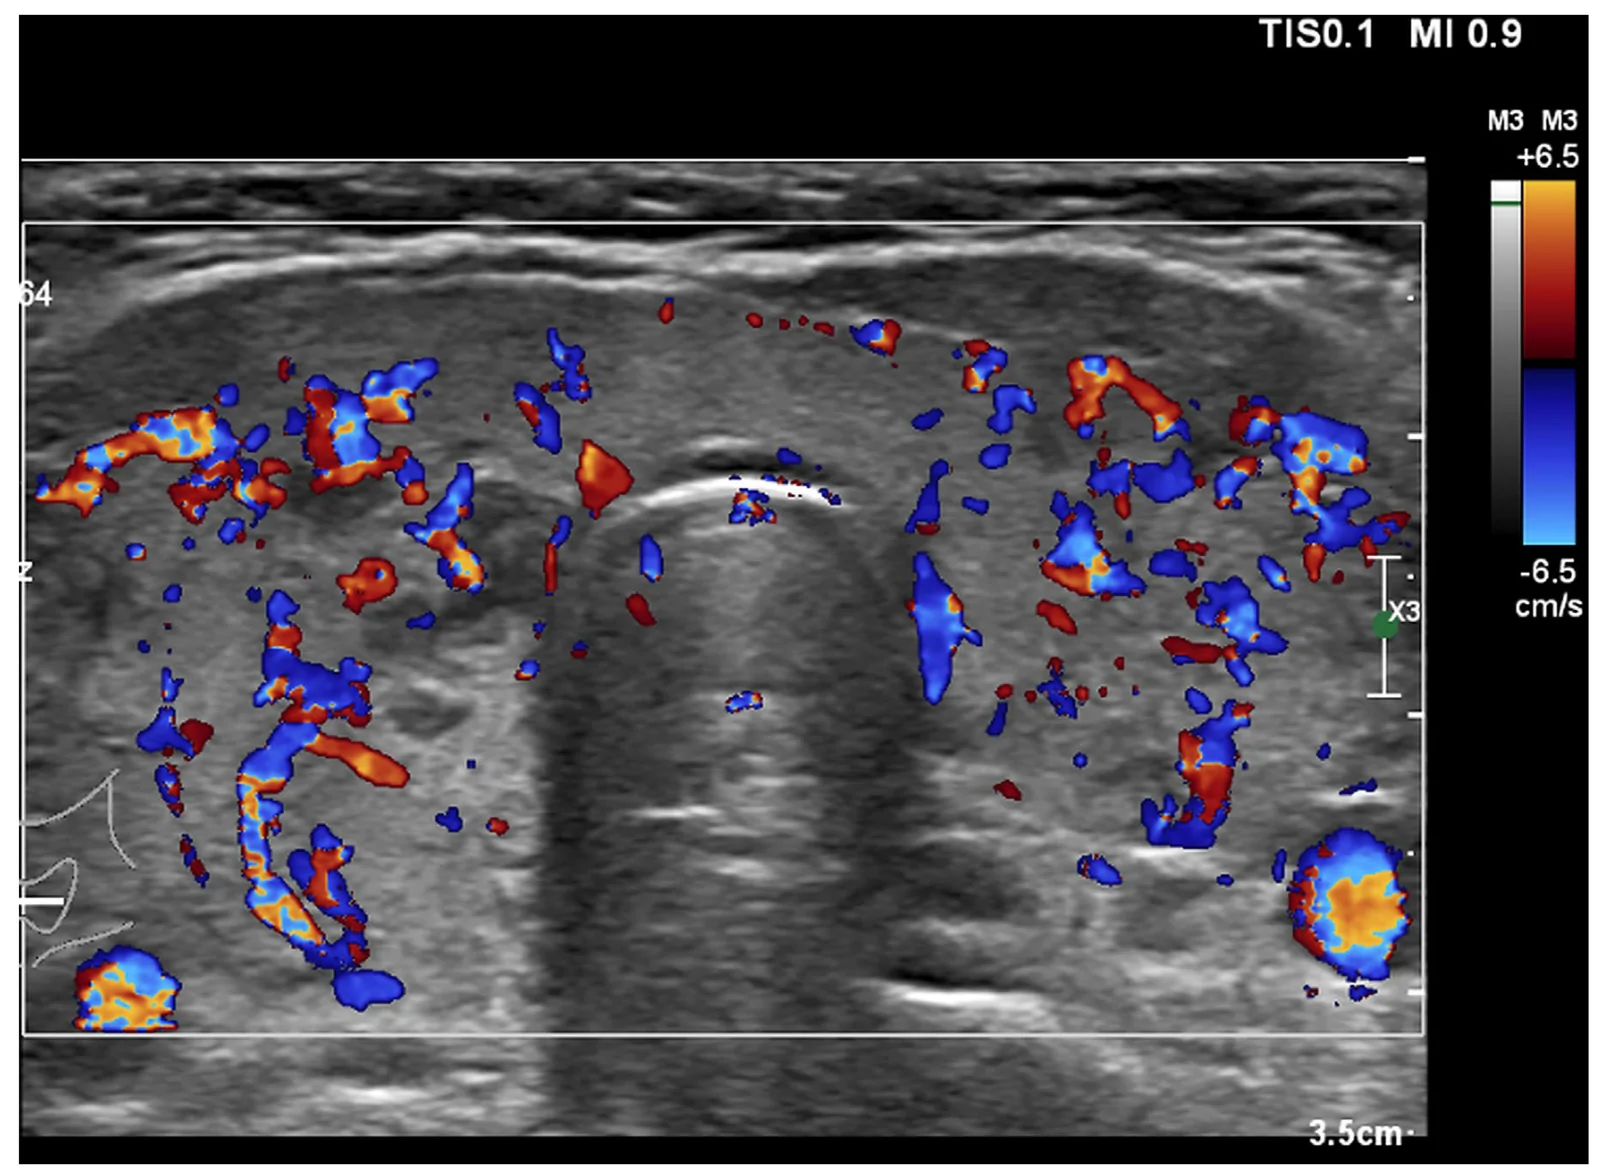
Thyroid ultrasonography shows diffuse parenchymal change with heterogeneous echotexture; no discrete nodule was identified.
